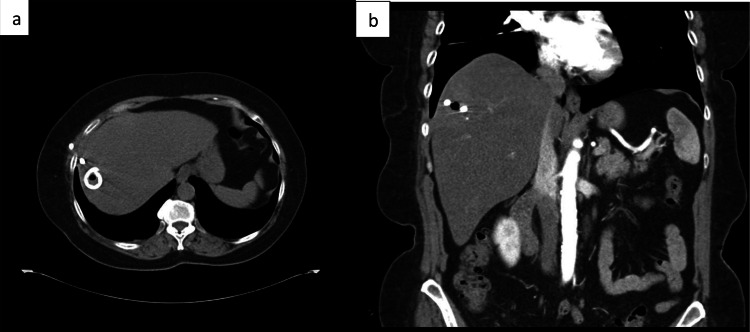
(a) Transverse section of the computed tomography (CT) abdomen and pelvis obtained at a follow-up visit is presented. This image demonstrates the presence of a Pigtail catheter in the right liver, with a small focal area of air within the hepatic parenchyma. No discernible residual drainable collection is identified at this time. (b) Sagittal section of the CT abdomen and pelvis, with consistent findings. The white opaque object in the right lobe of the liver at the previous lesion site, suggests the Pigtail catheter with residual air and resolved a prior liver abscess.
